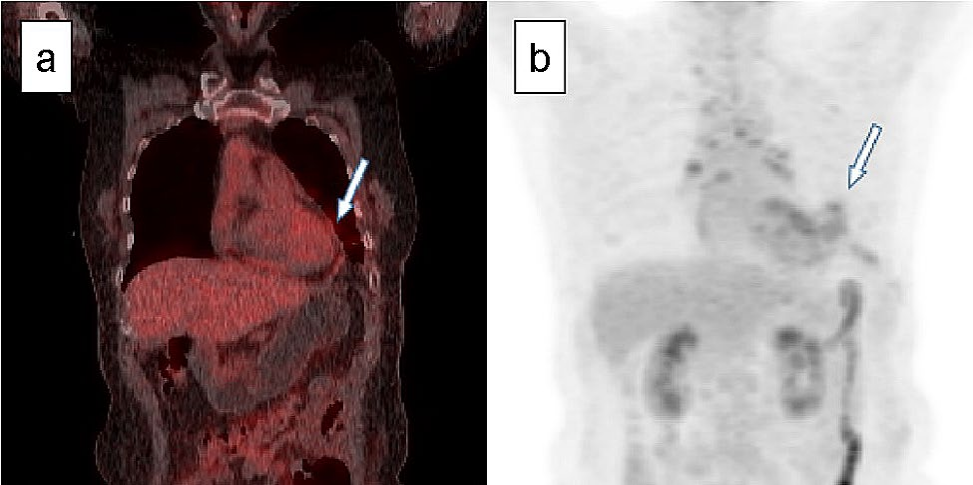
^18^F-FDG-PET/CT image before glucocorticoid treatment. (a) ^18^F-FDG-PET/CT scan showing increased ^18^F-FDG uptake in the pericardium (SUVmax = 2.9), indicating active inflammation (arrow). (b) ^18^F-FDG uptake in the pericardium (arrow) in the PET image. ^18^F-FDG-PET/CT: fluorine-18 fluorodeoxyglucose -positron emission tomography/computed tomography, SUVmax: maximum standardized uptake value
